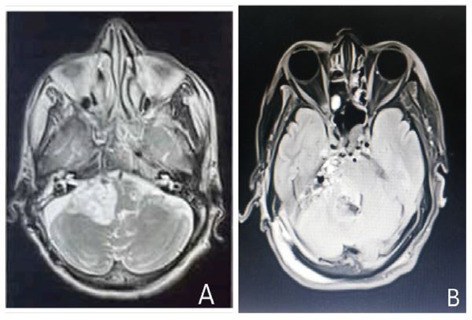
A. Preoperative MRI brain revealing a hyperintense lesion on T2-weighted image on axial plane measuring 4.2 cm × 4.0cm × 1.4cm. B. Postoperative MRI brain revealing heterogenous collection at tumor resection bed in right lateral aspect of posterior fossa where central component of the collection displays mixed signal.
